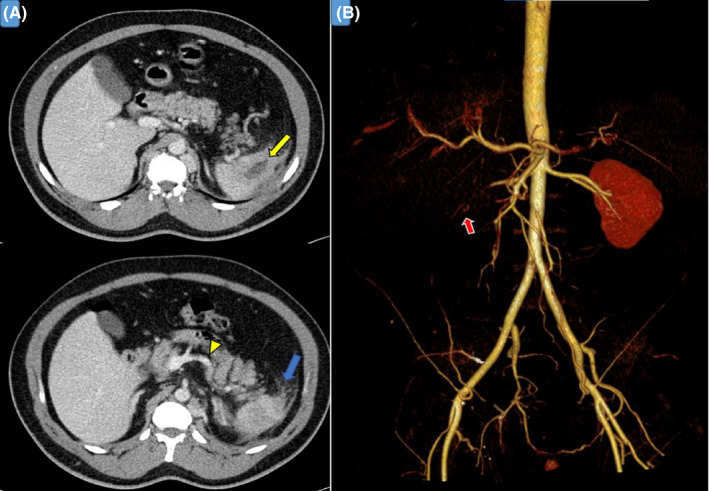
Images from Abdominal CT scan with contrast and 3D reformats. (A) shows splenic hypodense lesion (yellow arrow), which suggests splenic infarct with peri splenic fat stranding (blue arrow) despite normal splenic artery patency (yellow arrowhead). (B) demonstrates the absence of renal perfusion (red arrow) due to nearly total right renal artery occlusion
